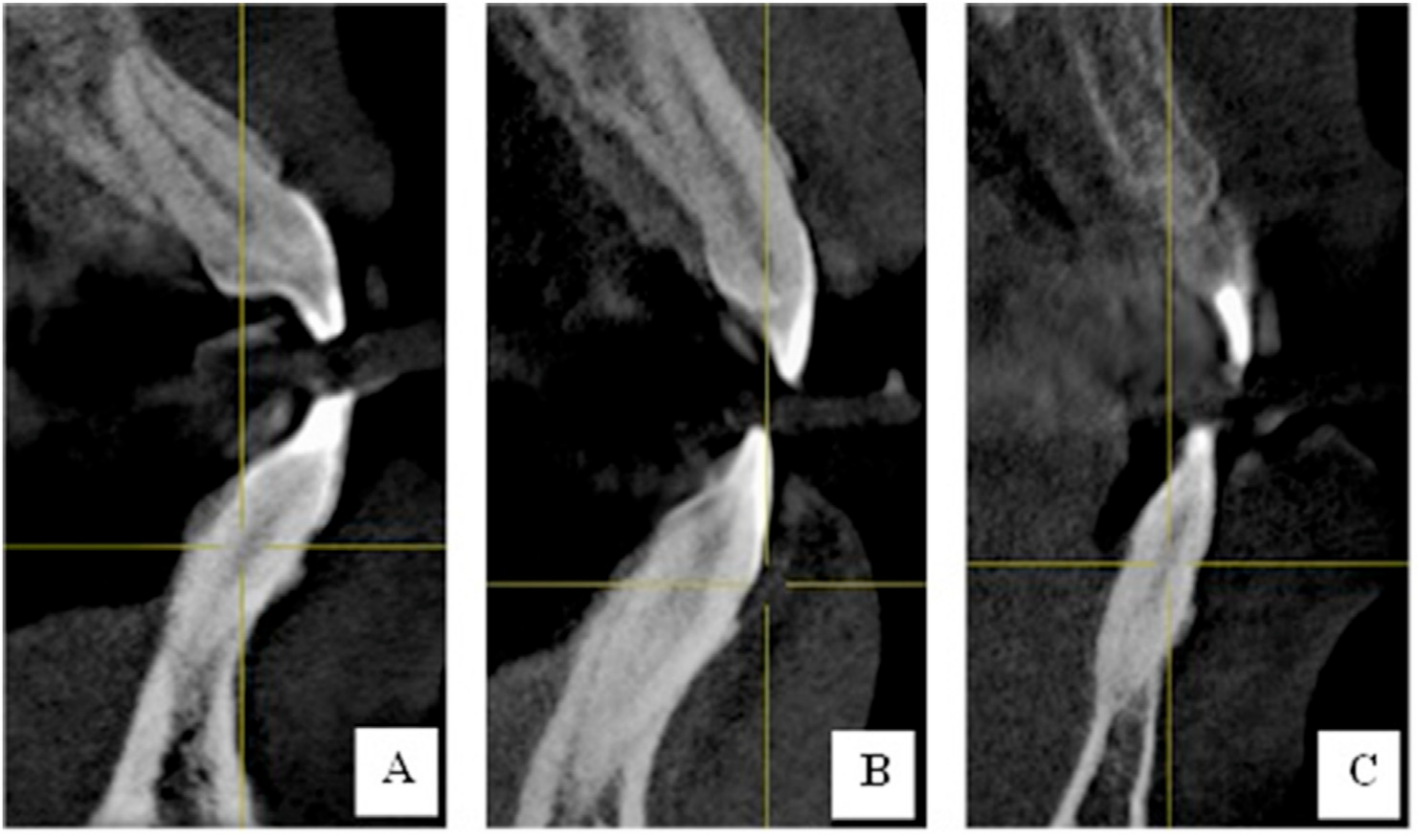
CBCT coronal section of mandibular central incisor (A: Type I; B: Type II; and Type III Vertucci's classification).
